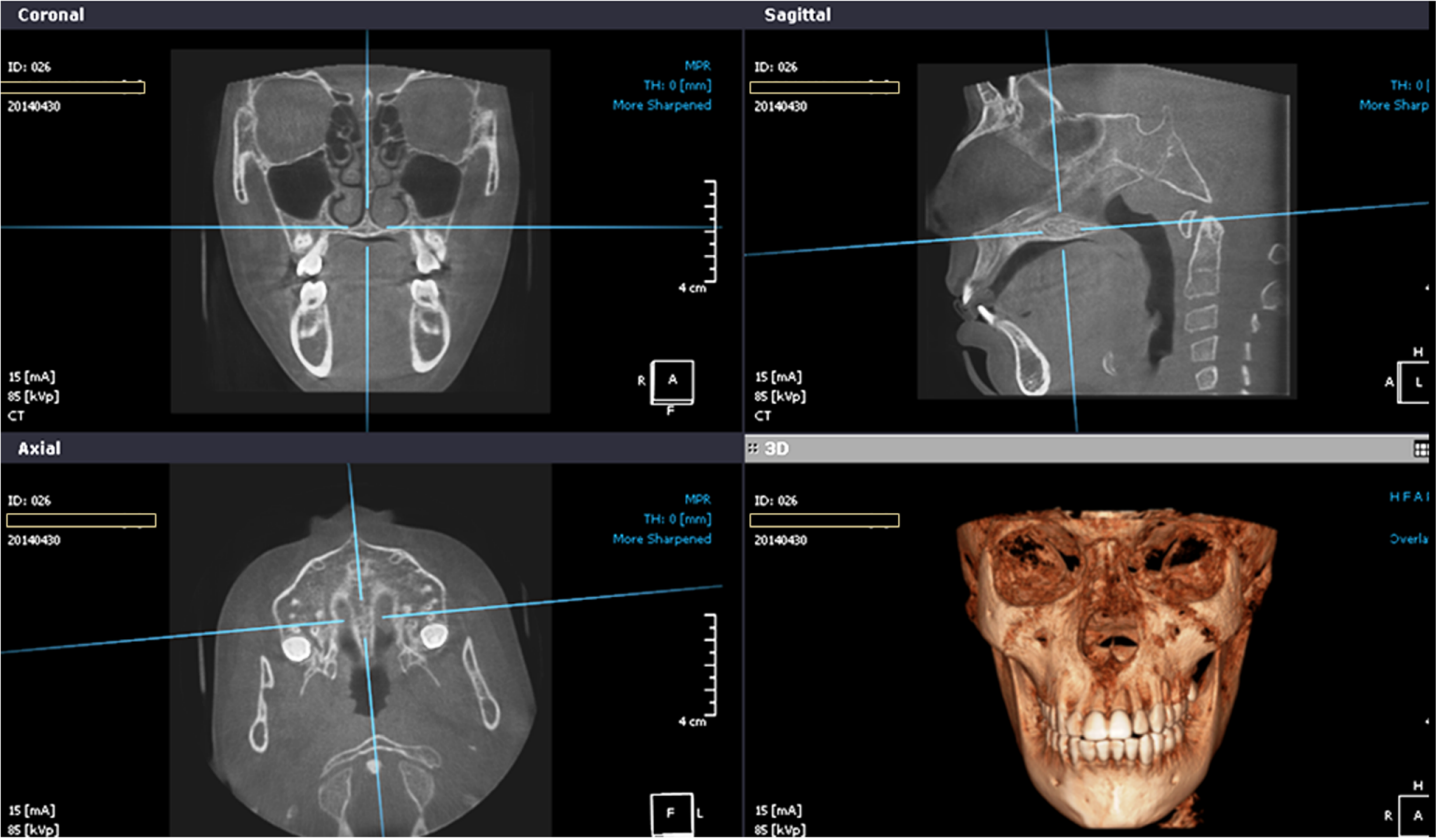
Standardization of CBCT radiograph in axial and sagittal planes
